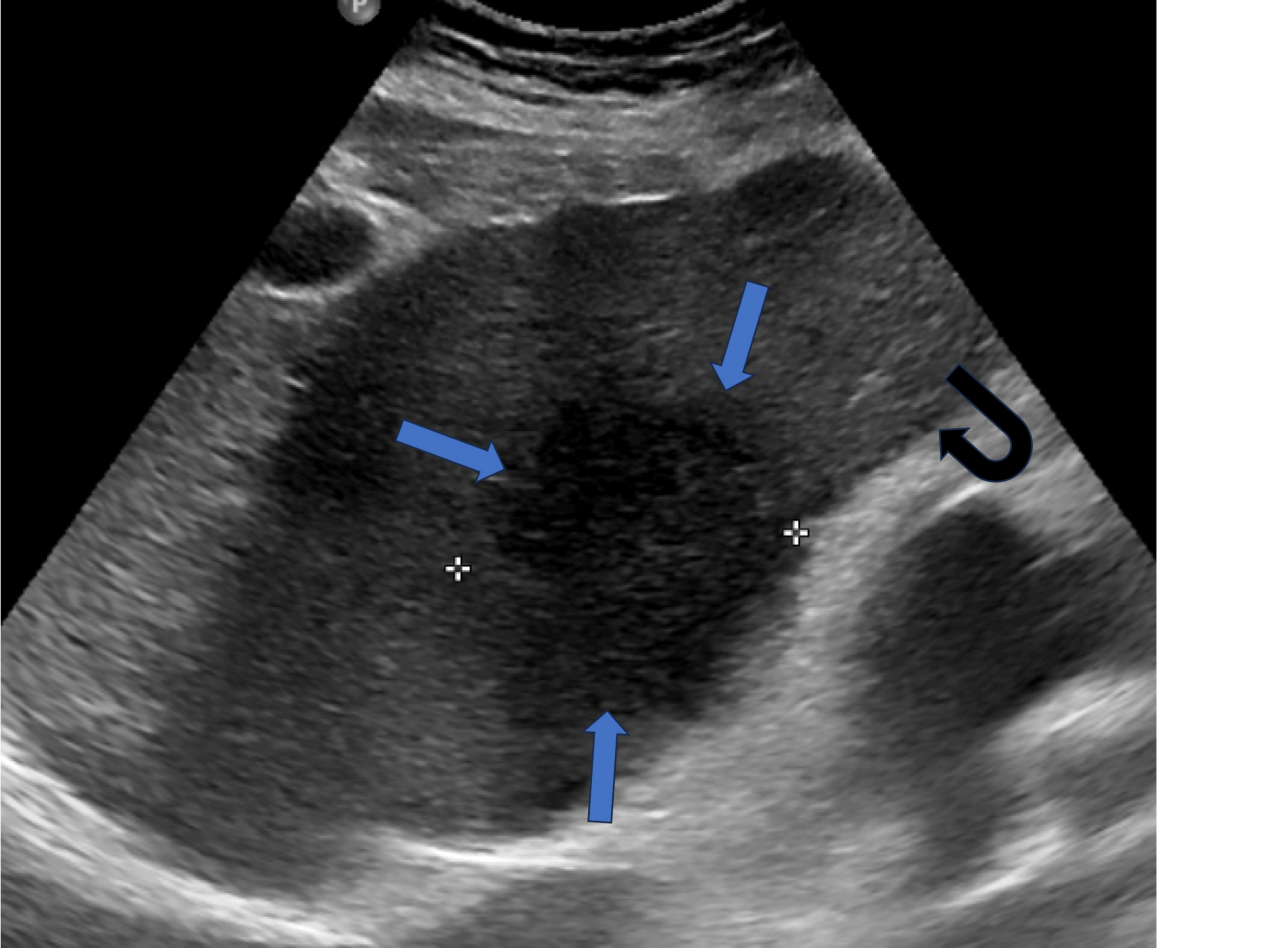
Transabdominal ultrasound of the abdomen demonstrating a hypoechoic lesion in the left lobe (blue arrows), suspicious of a malignancy The nodular liver margins represent cirrhosis (black curved arrow).
